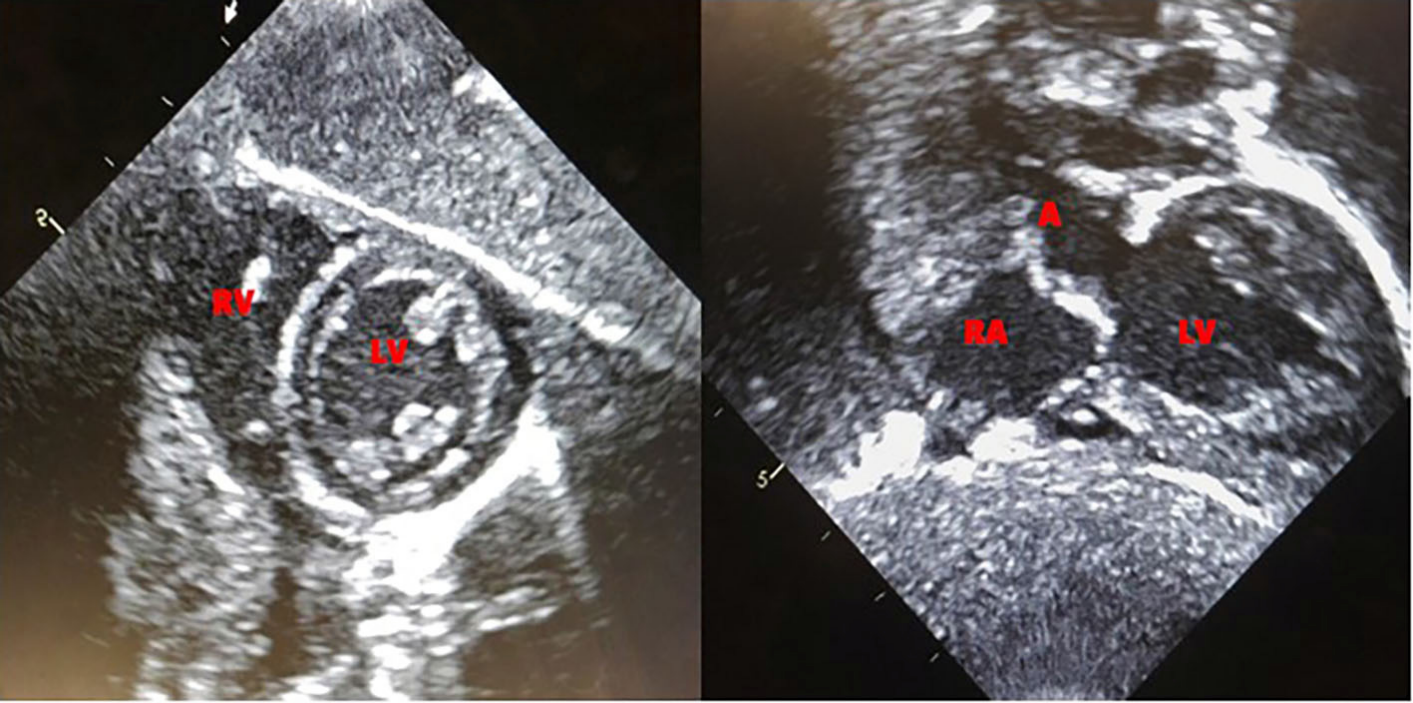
Echocardiogram at 11-weeks of life, showing resolution of ventricular hypertrophy.
